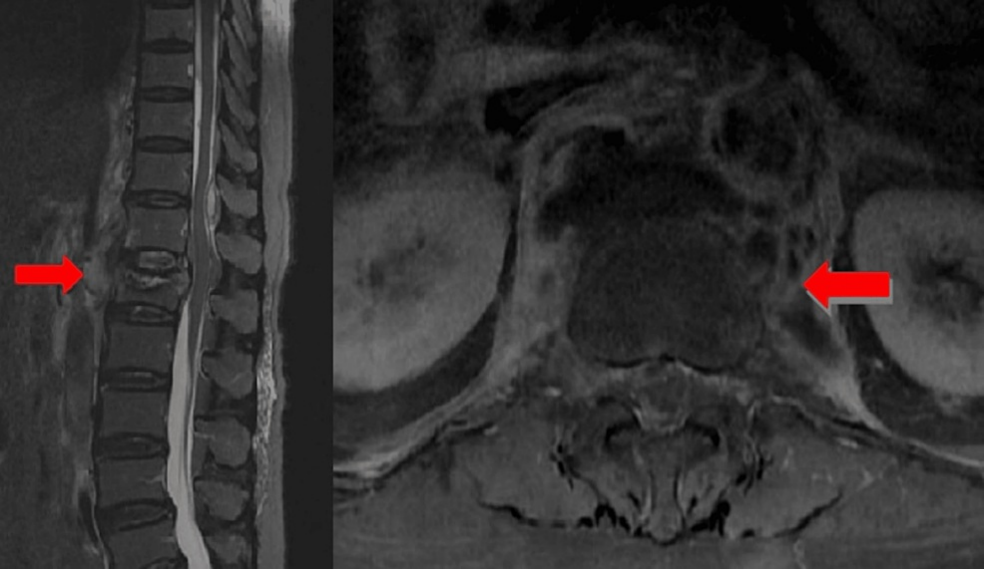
T2 weighted, fat suppressed magnetic resonance images: prevertebral and paravertebral abscess at T10-L1 level (red arrows).
